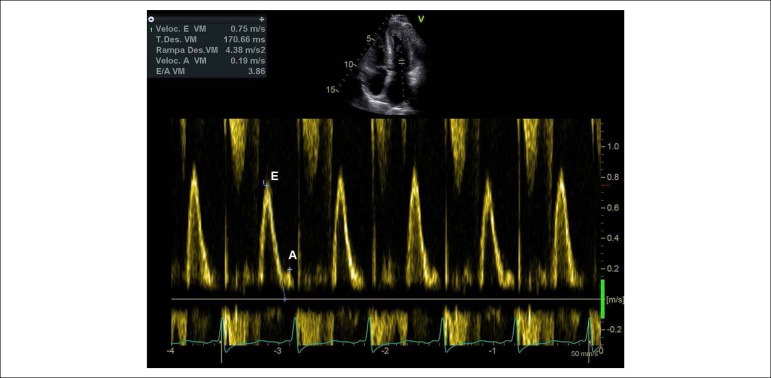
Image obtained from transthoracic echocardiography. Mitral Doppler spectral curve of a patient with amyloidosis showing a restrictive diastolic pattern (E/A ratio > 2)
